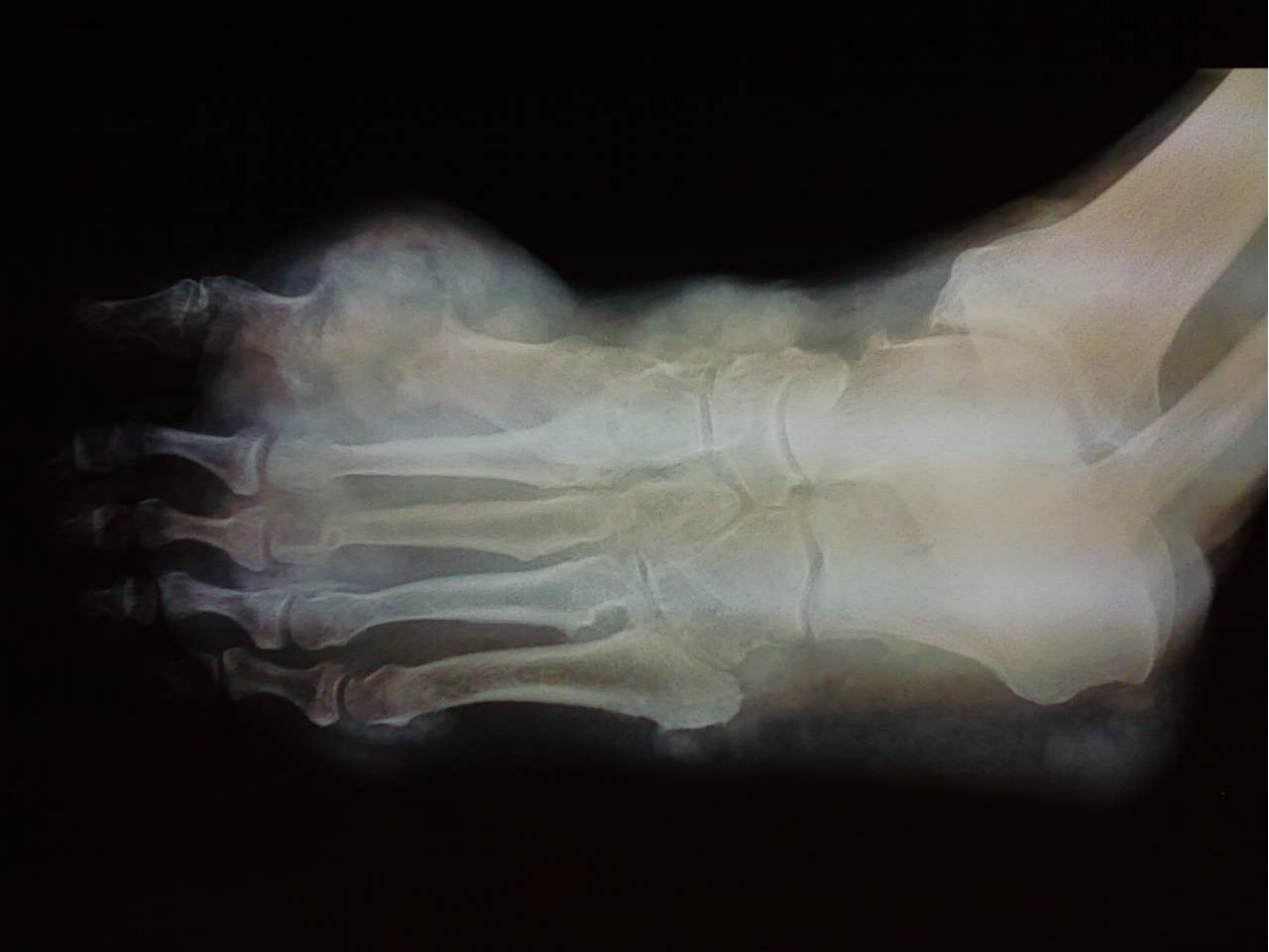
Radiographs of the foot. Total destruction of the first metatarsophalangeal joint and soft tissue swelling is shown as is focal involvement of dorsal and plantar surface of the foot (panniculitis).
